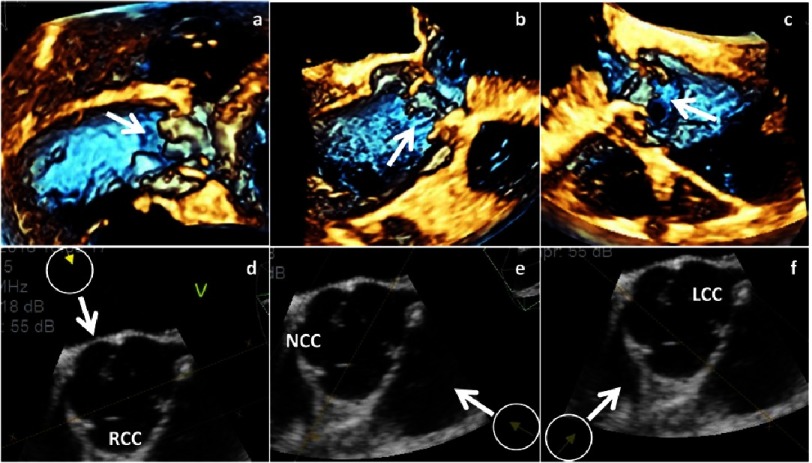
Fenestrations at the margins of the RCC (a), the NCC (b) and the LCC (c) are shown by 3D transthoracic echocardiography. The inhomogeneities of the cusp margin are labeled by white arrows. Below the orientation of the views are given by arrows in corresponding 2D short axis views (d-f).
